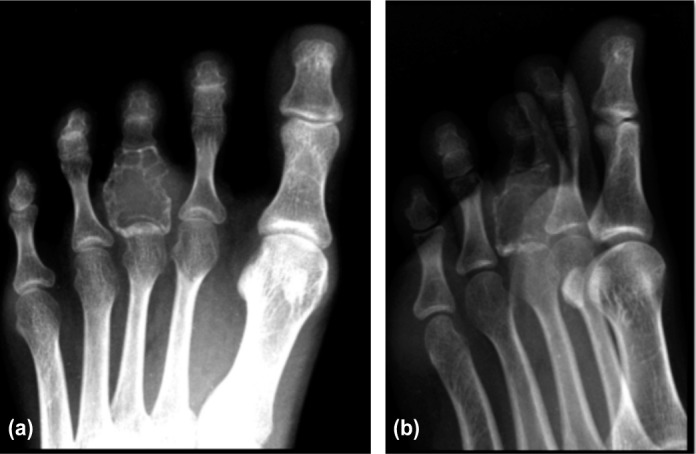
Left foot radiograph shows expansile lytic bone lesion in the proximal phalanx of left third toe. Multiple septations can be seen within the lesion. The cortical margin of the bone remains intact.
